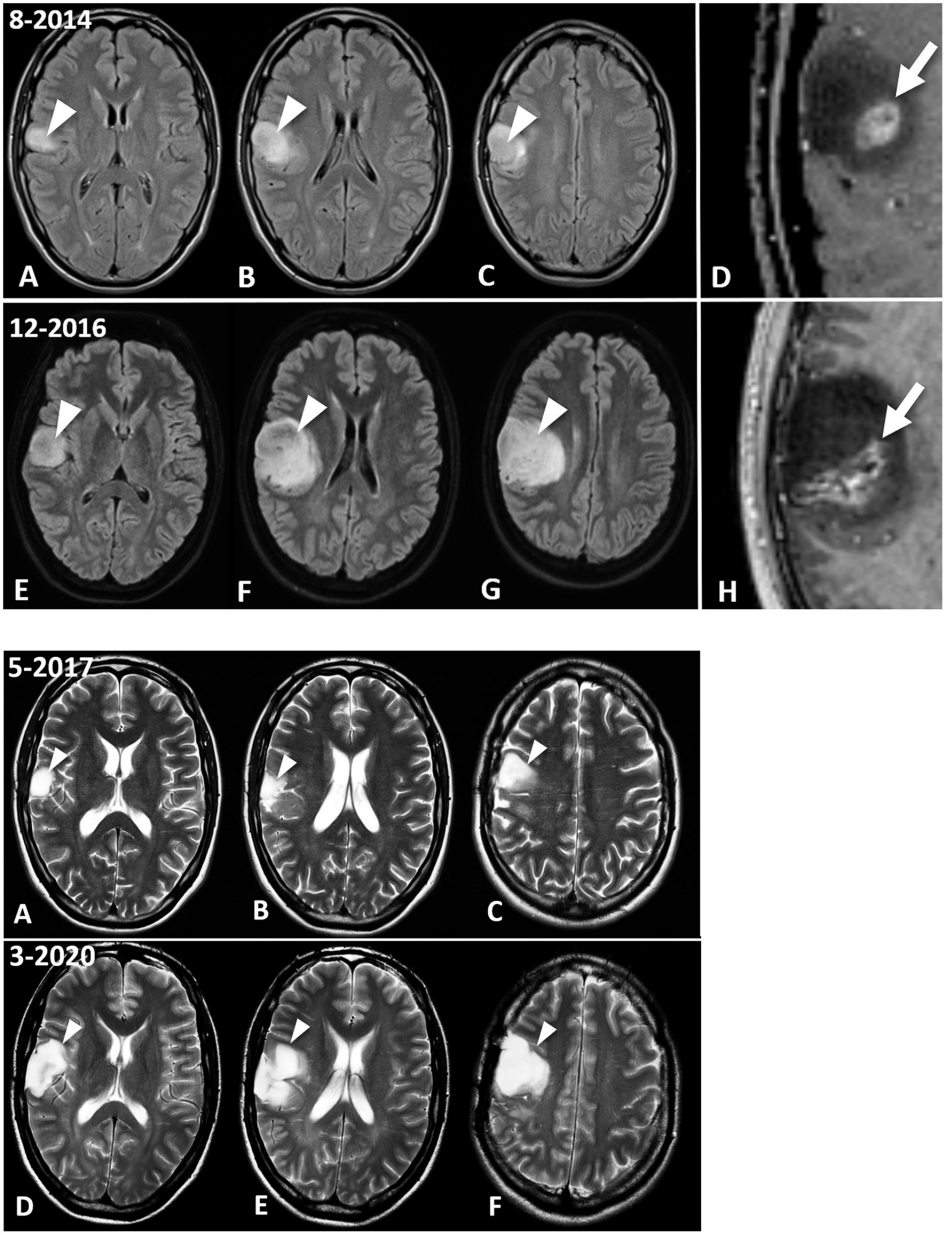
MRI images of the patient's brain tumour. Panel 1. Fluid Attenuated Inversion Recovery (FLAIR) images show the *IDH1*-mutant GBM at diagnosis in August 2014 (A–D) and ~8 months later, at the last follow-up MRI examination (December 2016) prior to surgical excision of the enhancing nodule (E–H). The lesion discovered on August 2018 is centered in the right inferior frontal lobe (A,B, arrowheads), and abuts the premotor cortex (C, arrowhead). There is an enhancing nodule within the lesion, as seen in the magnified post-contrast image (D) (arrow). This enhancing lesion measured 1.25 mL, which is calculated using the V = ABC/2 formula. Follow-up MRI (E–H) demonstrates interval progression of the non-enhancing tumor (arrowheads). Interval increase in size of the enhancing lesion, measuring 5.97 mL, was also observed (H, arrow). Panel 2 shows the evolution of the lesion between the May 2017 surgical excision (A–C), and in the most recent MRI evaluation, dated March 2020 (D–F). T2-weighted images indicate the residual GBM (arrowheads). (B) shows the T2-hyperintense GBM filling the surgical cavity. The lesion in the premotor cortex is clearly seen in (C). Please note that the surgical debulking involved only the enhancing tumor, while the largest non-enhancing part of the GBM was not excised by the neurosurgeon. The most recent brain MRI (D–F) shows interval increase in size of the GBM (arrowheads), which remains circumscribed to the right frontal region without infiltrating the white matter tracts.
